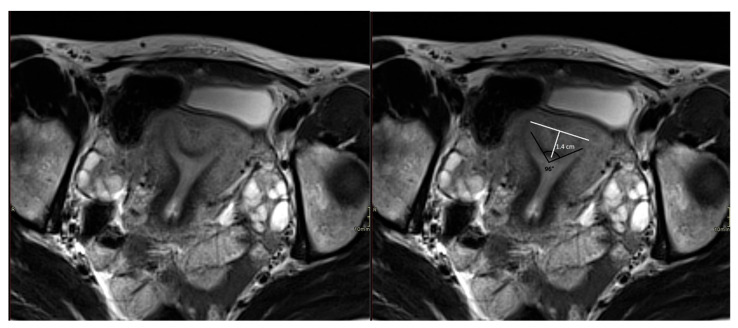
30-year-old female with on ultrasound suspicion of a uterine septum. Axial T2-weighted MR image parallel with the long axis of the uterus. The external uterine contour is convex, and the myometrium is thickened at the level of the fundus, suggestive of a partial uterine septum. The indentation depth is 1.4 cm, and the septum angle is 96°. According to the AFS classification this is a partial septate uterus. In the ESHRE/ESGE classification this is U2a and in CUME the criteria are fulfilled for a septate uterus. In the ASRM classification, however, this Müllerian duct anomaly is indeterminate because the septum has a length of >1 cm and an angle of >90°, excluding the diagnosis of septate uterus (the septum angle should be <90° in that case) as well as normal/arcuate uterus (the septum length should be <1 cm in that case).
